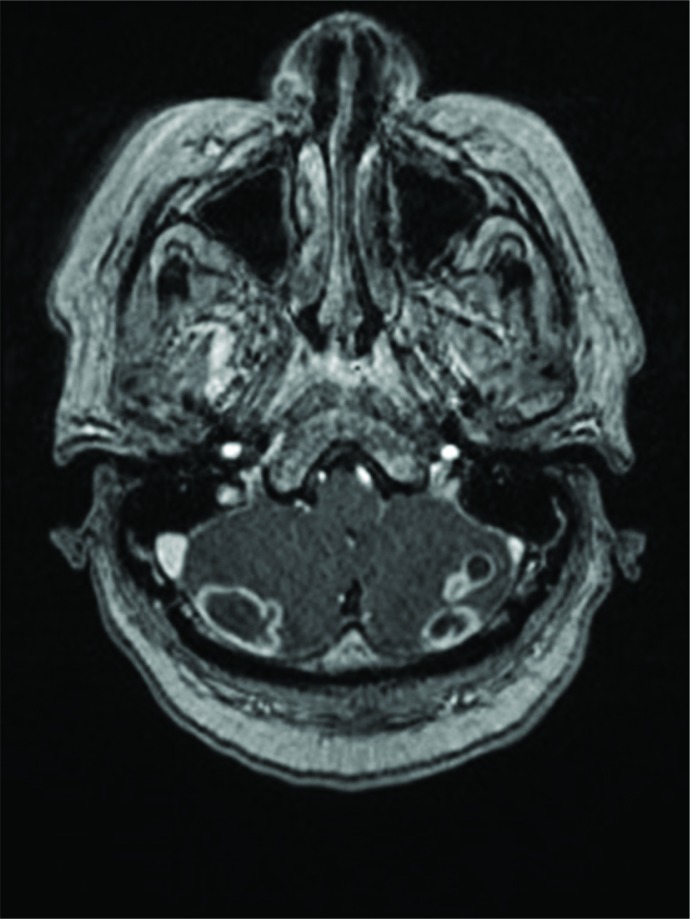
MRI of patient showing multiple ring-enhanced lesions in the cerebellum.
